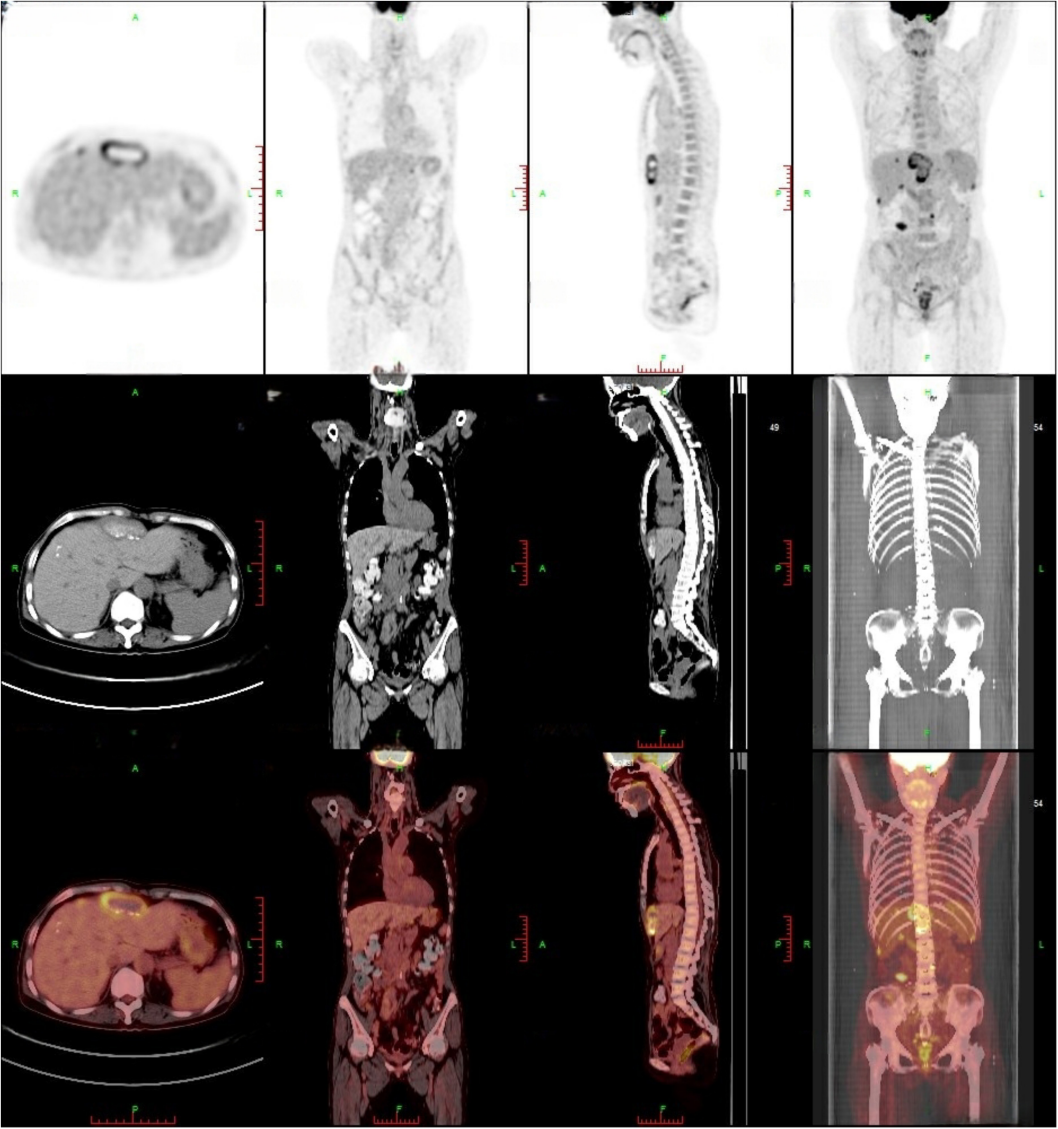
PET-CT findings: multiple peritoneal nodules and masses (approximately 5.5 × 2.2 cm) exhibited increased FDG accumulation, with a maximum SUV of 7.1, suggesting peritoneal metastasis. A high-uptake lesion beneath the right abdominal wall was recommended for percutaneous biopsy.
